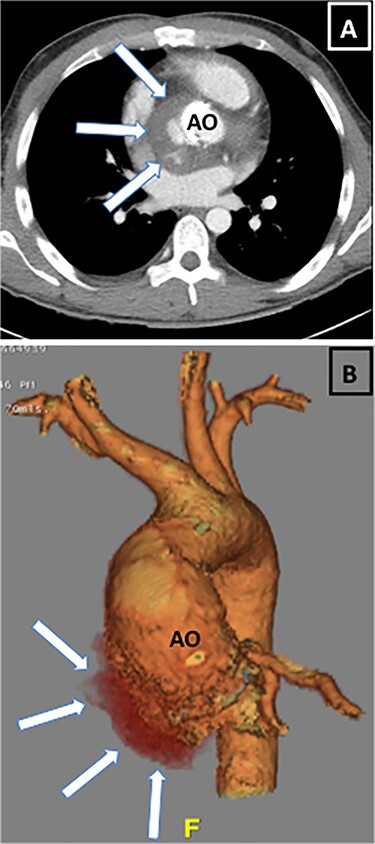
White arrows on CT showing expanding aortic root abscess (A) short-axis view, (B) rendered reconstruction. AO = Aorta.
